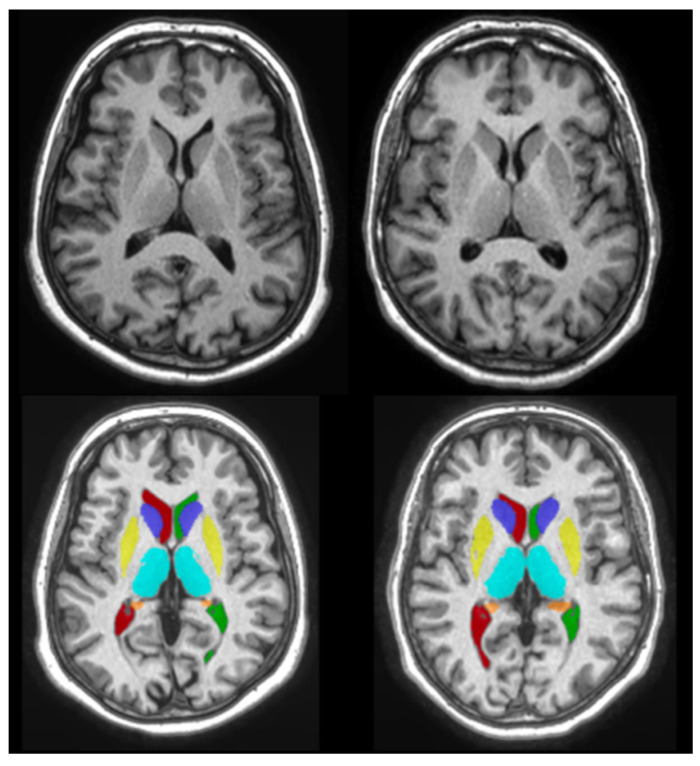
The 3D T1-weighted magnetic resonance images of 68-year-old dizygotic twins taken at the Medical Imaging Centre, Semmelweis University. The images were segmented using volBrain. The subcortical structures were delineated and their volumes were calculated.
